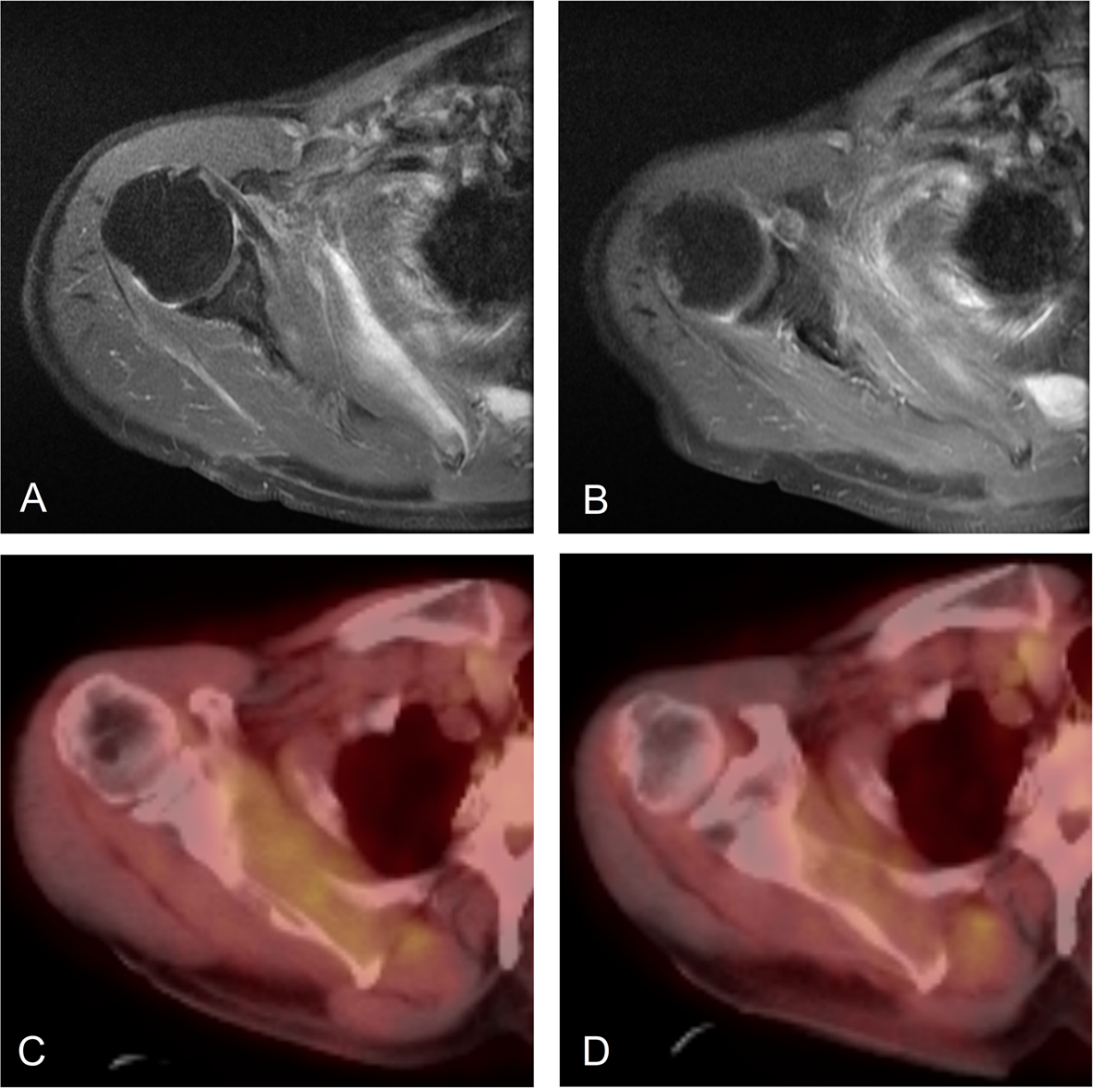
An undifferentiated sarcoma occurred in the chest wall of a 74-year-old male. A small enhanced lesion persisted three months after CIRT (a), and the size of the lesion increased on MRI performed five months after CIRT (b). FDG-PET/CT showed no change in the FDG accumulation between before (c) and after the recurrence (d)
